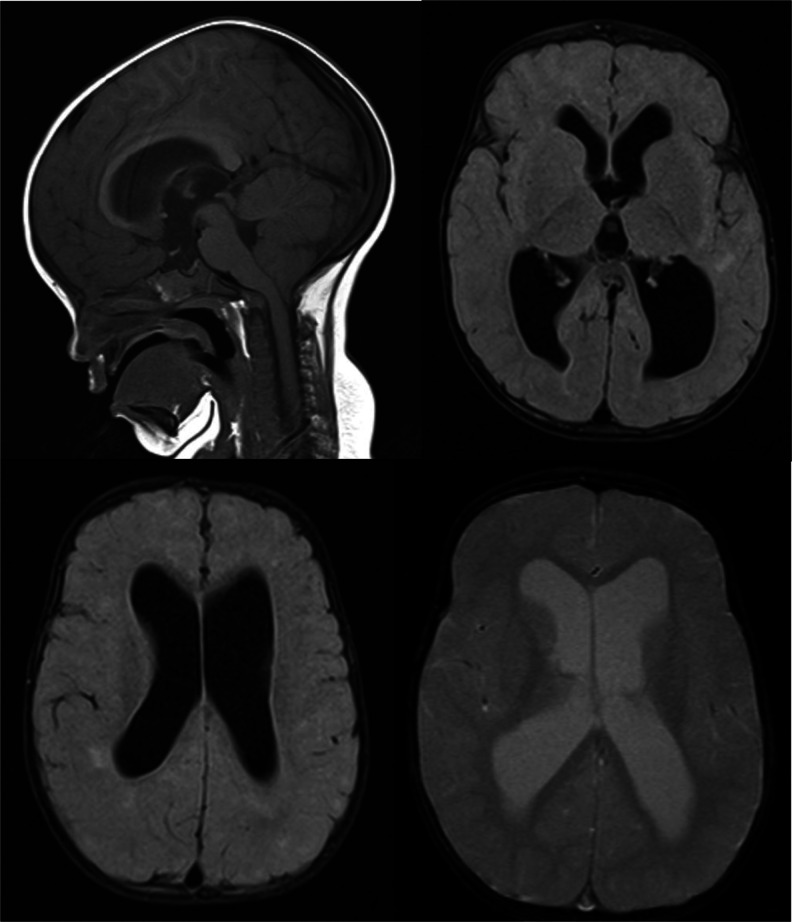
Magnetic resonance imaging (MRI) of patient 1 at the age of 3 years showing a Chiari malformation type 1, ventricular enlargement, cerebral subcortical atrophy, hyperintense periventricular lesions, and hypomyelinization
